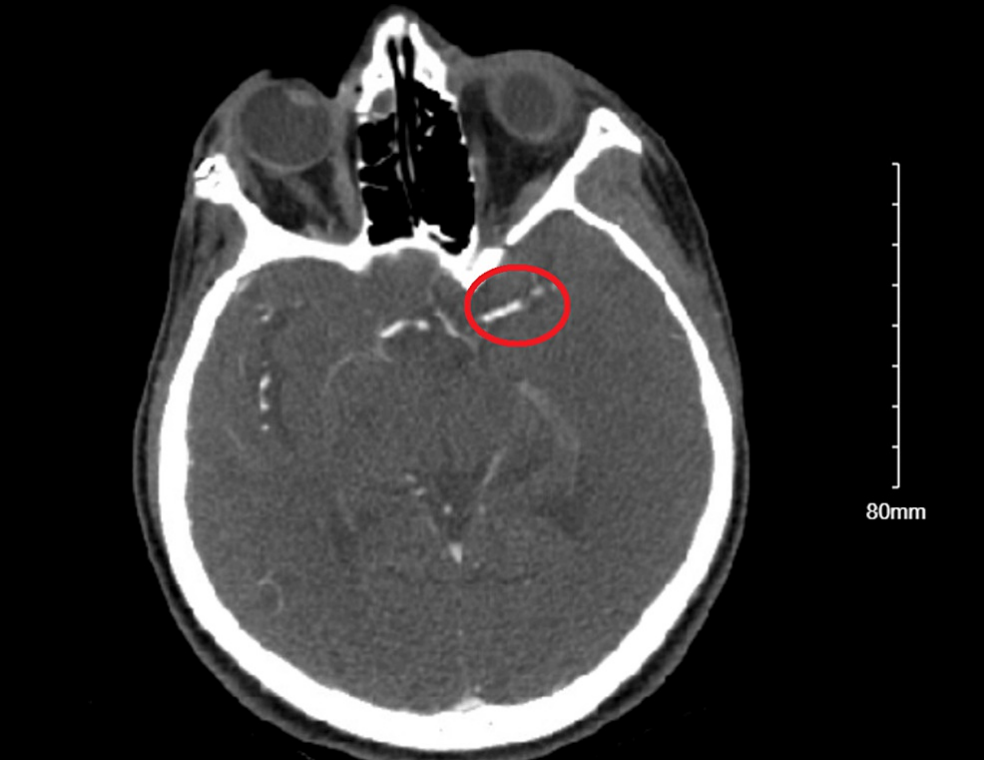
Computed tomography angiography showing occlusion of the left M1 division of the middle cerebral artery.
